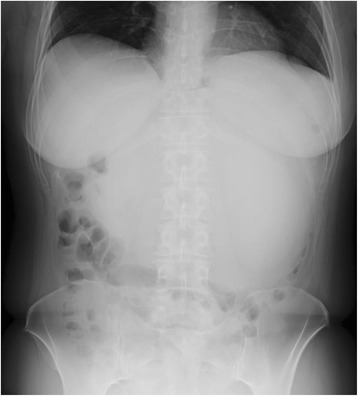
An abdominal X-ray showing a diffuse opaque area in the upper abdomen without a gastrointestinal gas shadow; this finding was accompanied by a downward dislocation of the transverse colon
